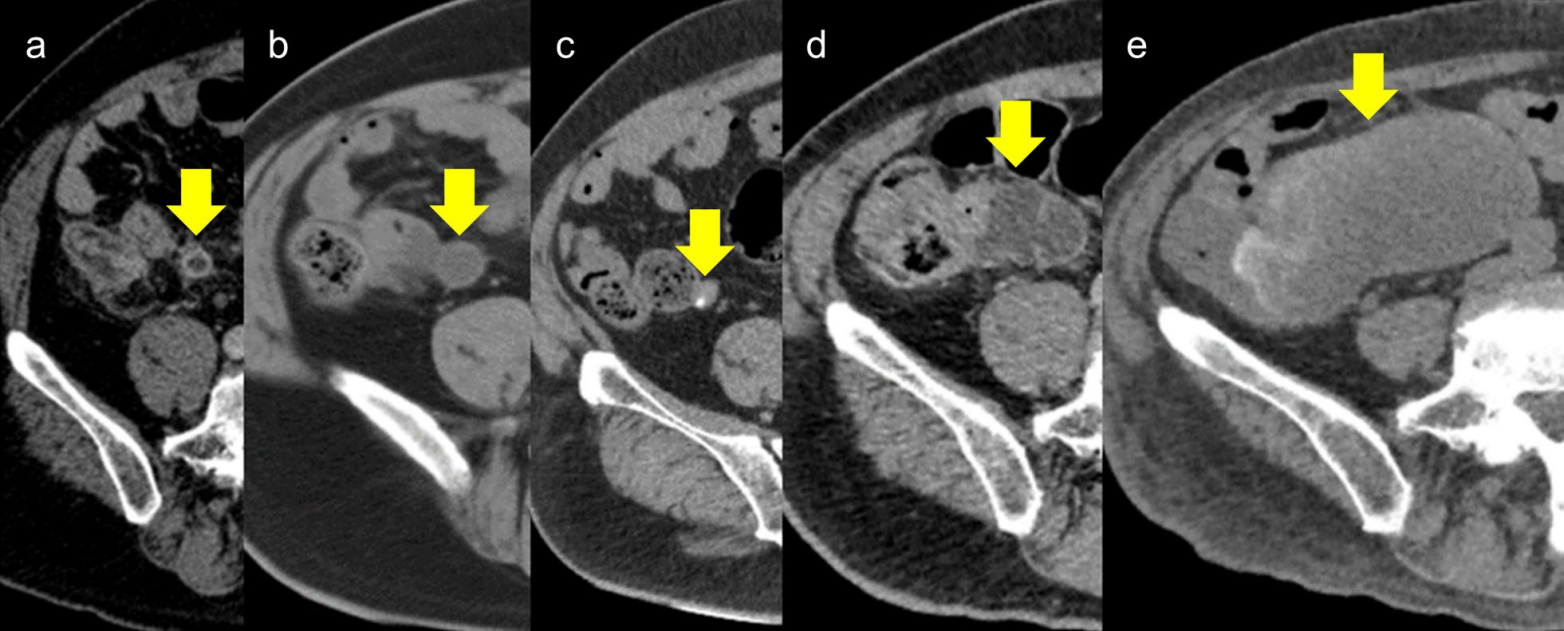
Retrospective review of the changes over time in the appendiceal tumor on CT images (Case 1). a The appendiceal tip could be identified just before appendectomy. b An appendiceal tip remnant was suspected one month after appendectomy (19 × 19 mm). c The tip remnant had shrunk six years after appendectomy (15 × 10 mm). d The tip remnant had enlarged and formed a cyst seven years after appendectomy (35 × 25 mm). e The cystic lesion had grown more 12 years after appendectomy (92 × 45 mm)
